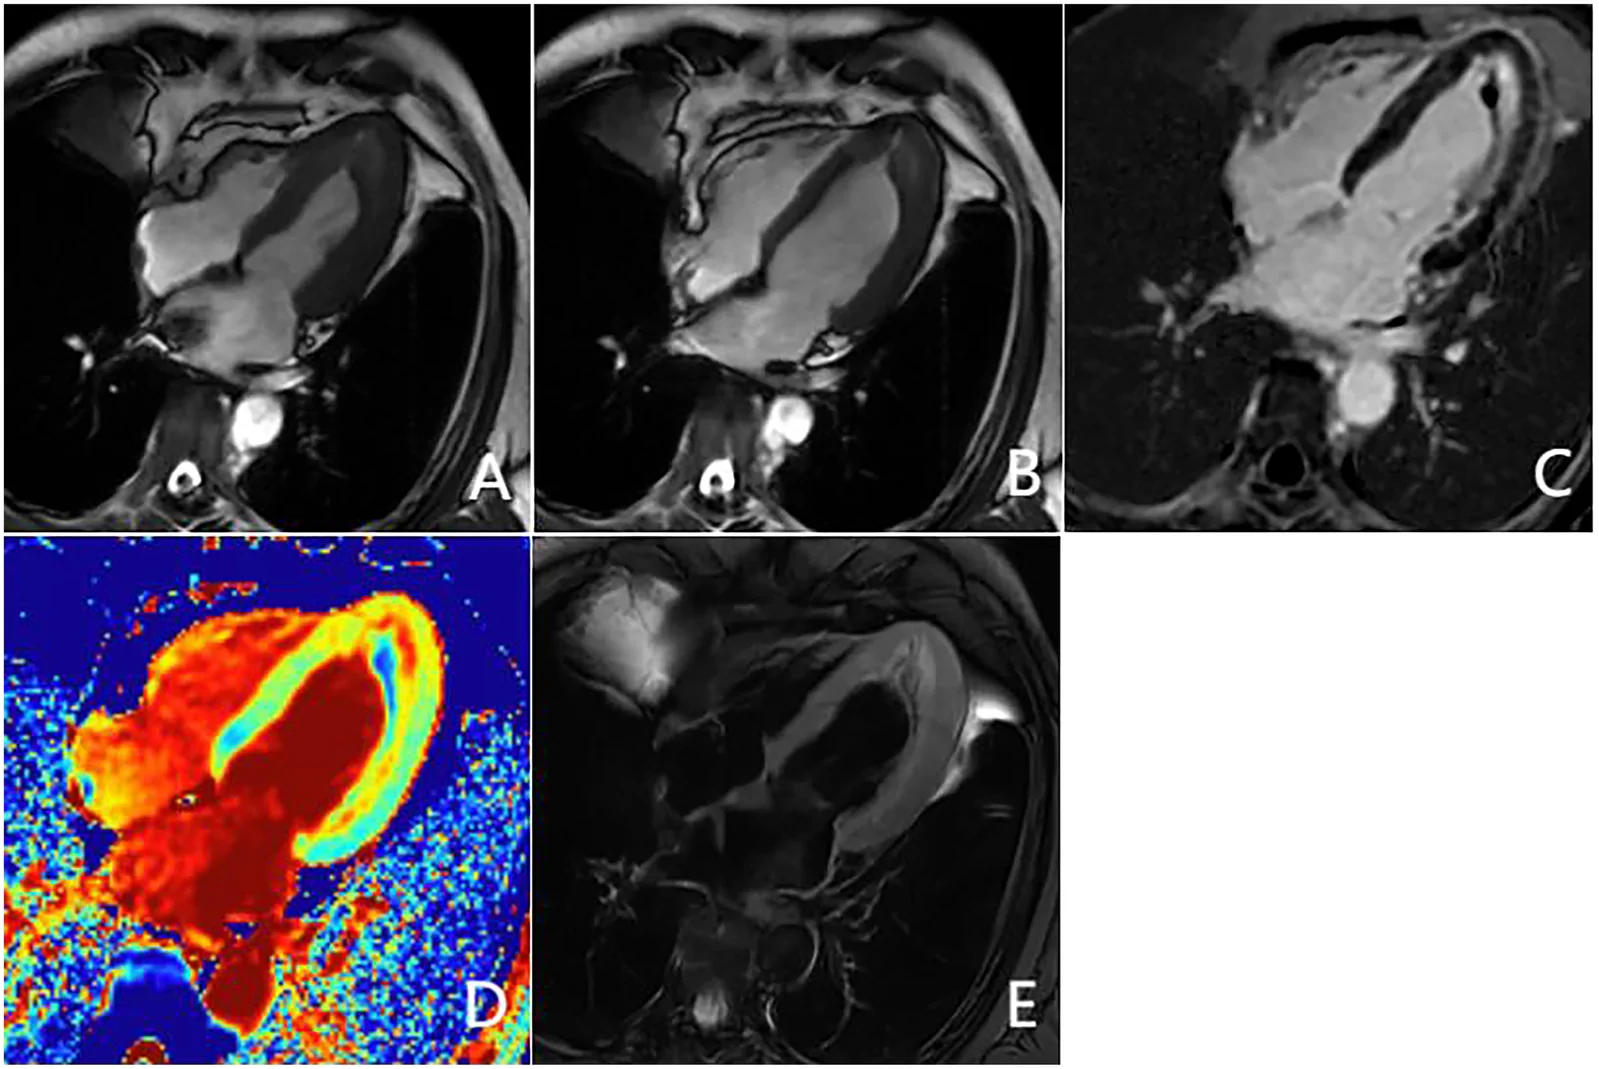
Cardiac magnetic resonance findings of Löffler endocarditis with mural thrombus. (A,B) Four-chamber cine images in systole and diastole show abnormal left ventricular endocardial thickening and an apical intracavitary filling defect. (C) Phase-sensitive inversion recovery late gadolinium enhancement imaging shows subendocardial enhancement with an adjacent non-enhancing intracavitary layer representing mural thrombus. (D) T1 mapping demonstrates abnormal signal characteristics in the involved endomyocardium. (E) Fat-suppressed T2-weighted imaging shows increased signal in the involved endomyocardial region, consistent with active edema or inflammation.
